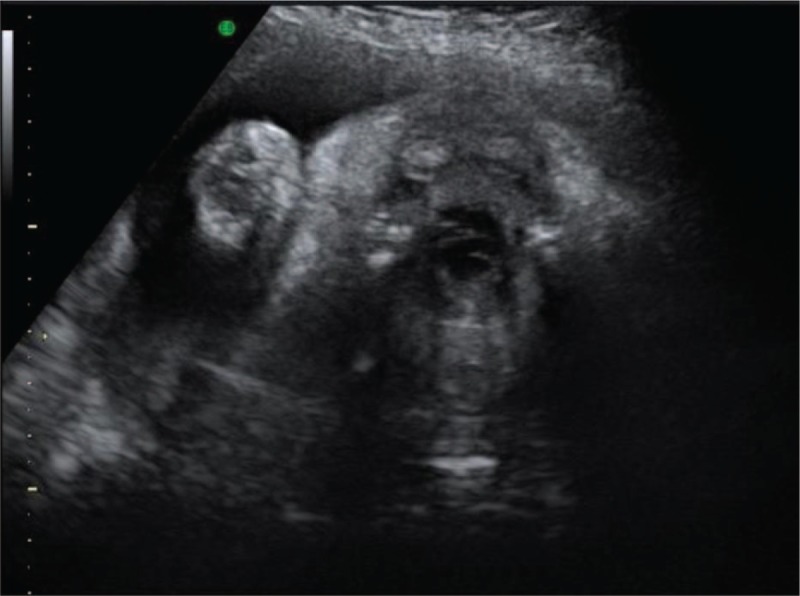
Follow-up ultrasound examination at 31 weeks of gestation. A tiny septum in the cyst can be seen.
